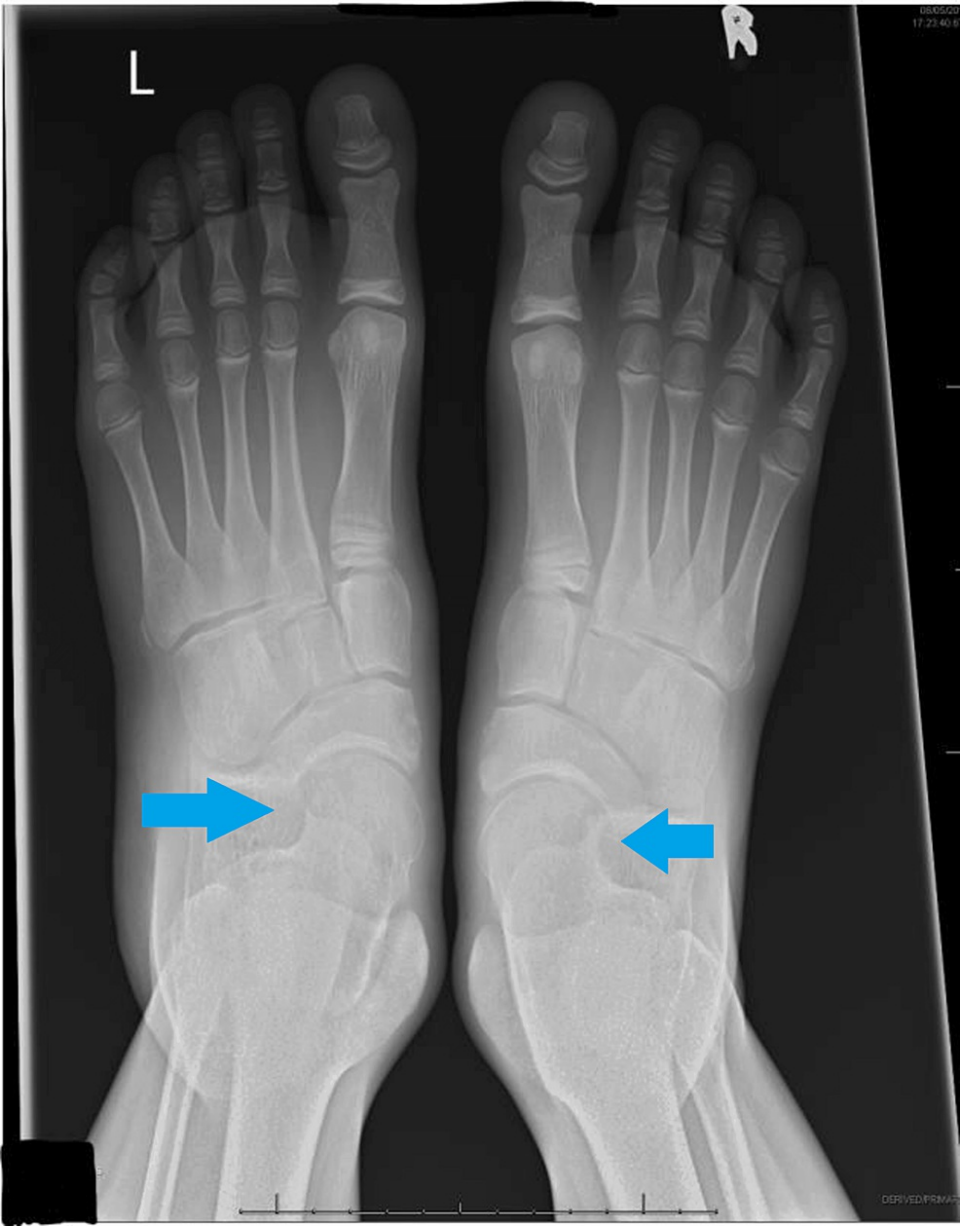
Radiograph of the bilateral calcaneonavicular coalition in the dorso pedal view. L: left; R: right
